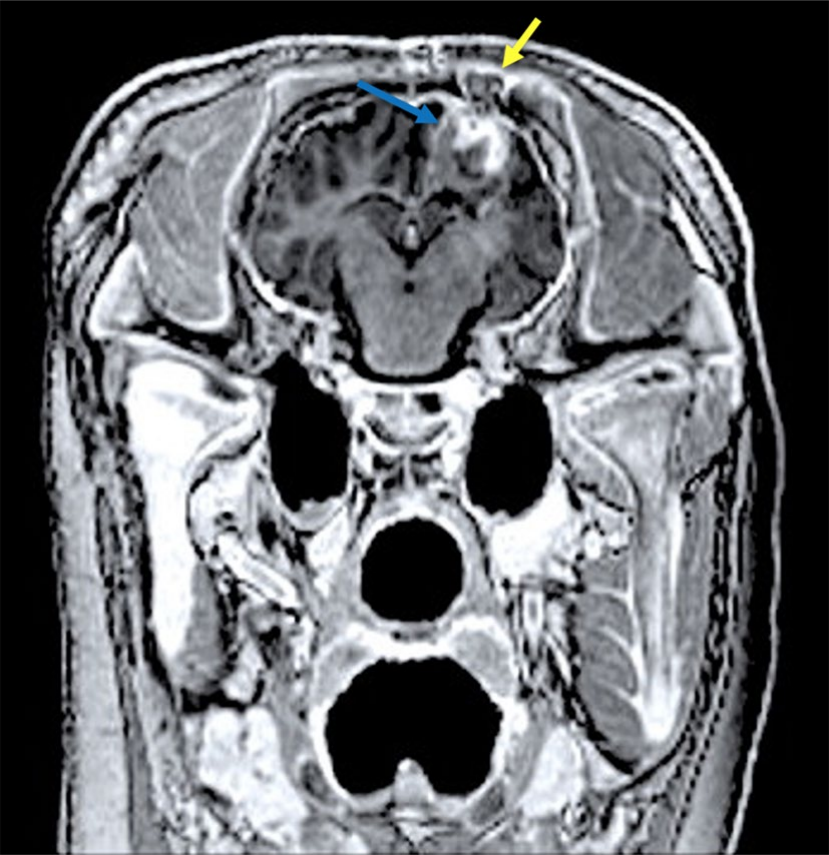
Postoperative T1 weighted MRI with contrast inversion prepared gradient echo coronal section of the brain and cryolesion. A large, well-defined lesion (blue arrow) is seen cranial to the right ventricle with a thin contrast enhancement peripherally. From the burr hole and into the cryolesion, a wedge-shaped lesion (yellow arrow) is seen, which is compatible with the spongostan used to close after the procedure.
